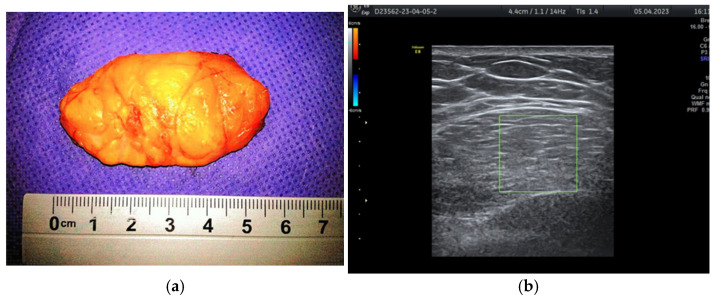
Breast lipoma in a 52-year-old woman (a) macroscopic pathological aspect; (b) ultrasound exam—hypoechoic aspect with thin striations.
